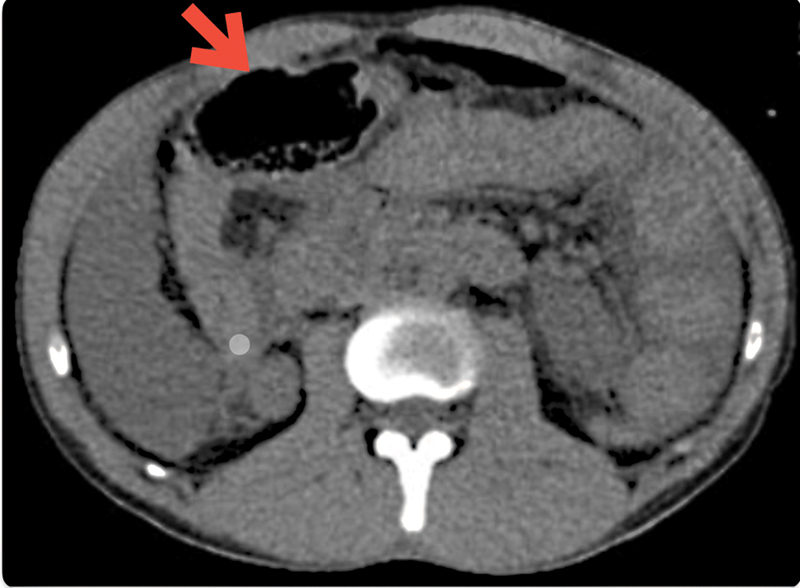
Abdominal computed tomography (CT) scan showing duodenal disruption with intestinal wall pneumatosis (the arrow signifies the duodenal disruption).
